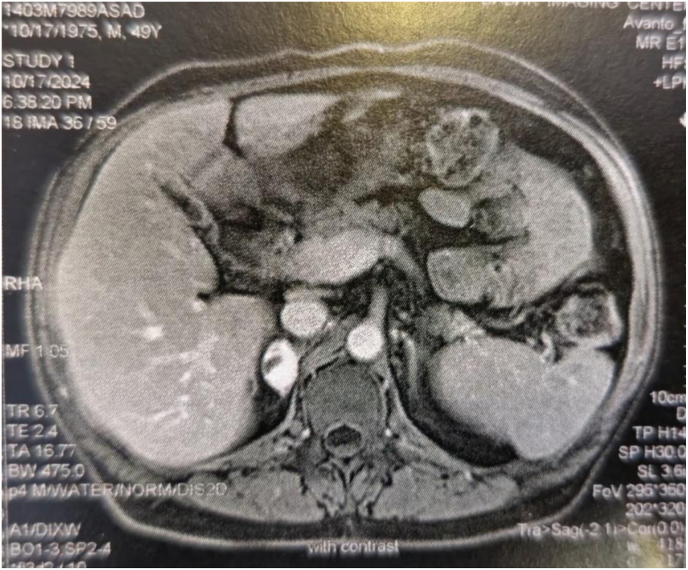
Contrast-enhanced CT demonstrated a hypervascular, heterogeneous mass in the right adrenal region without calcification, fat attenuation, or adjacent organ invasion.
